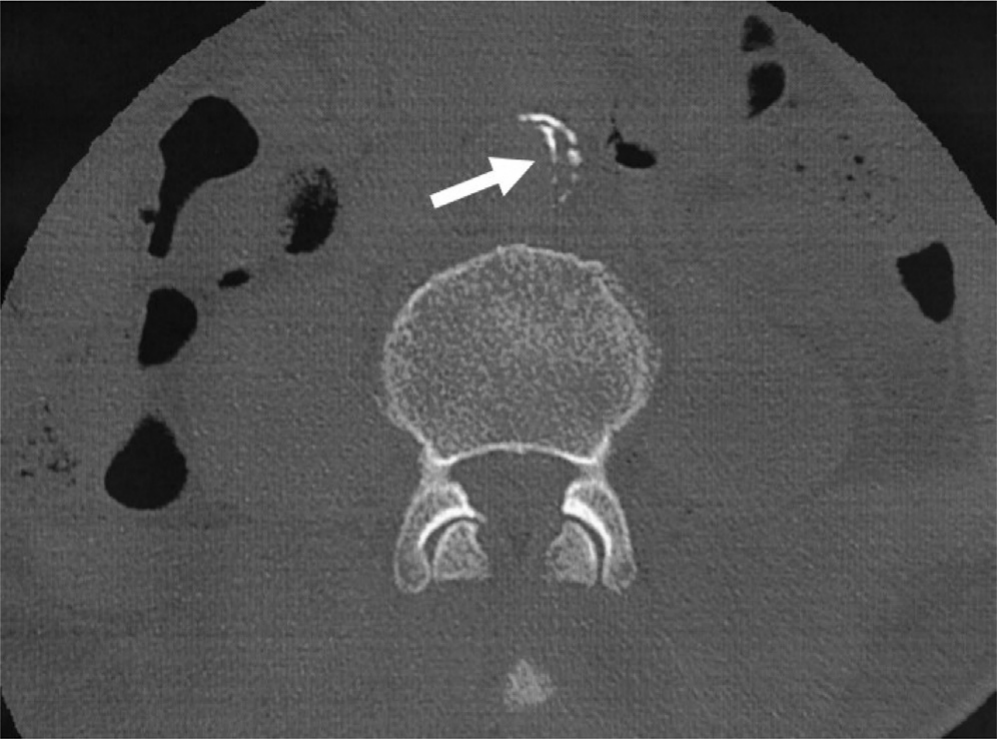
Inward displacement of intimal calcification (⇨) as indicated on the computed tomography (CT) scan performed by the patient's previous doctor. Findings suggested local abdominal aortic dissection.
